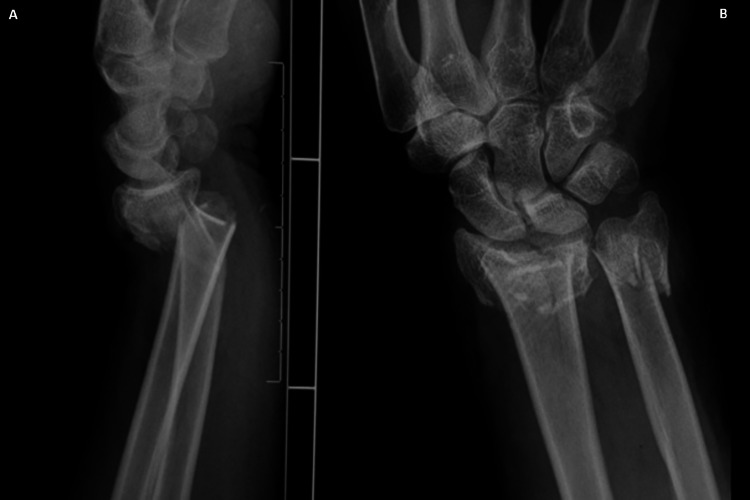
The fracture at the distal level. Anteroposterior (A) and lateral (B) radiographs showing distal radius and ulna metaphyseal comminuted fracture with dorsal angulation and displacement.
